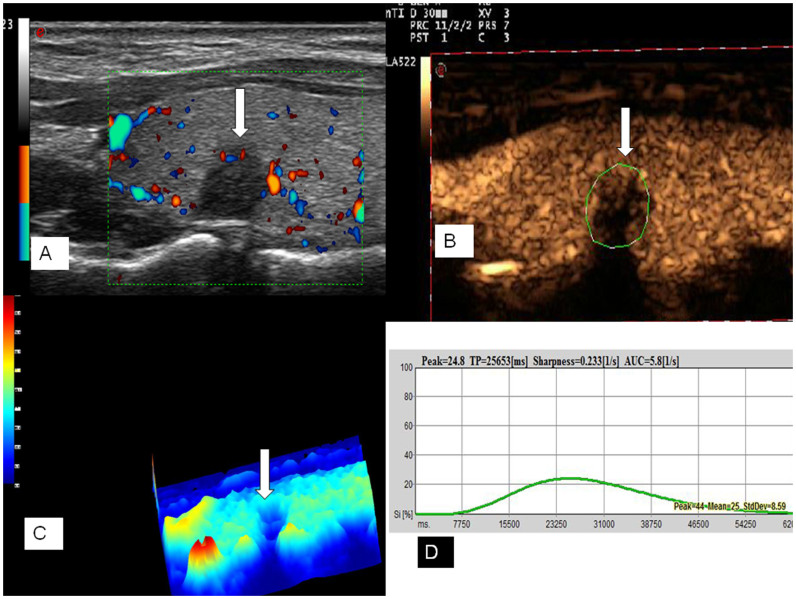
Ultrasound images of a 35-year-old female PTMC patient without CCLNM of the right thyroid lobe. Doppler image showed poor signals in the nodule (A). The CEUS image showed a poor and heterogeneous enhancement (arrow) in the nodule (B). Parametric map showed that the nodule was in blue (arrow), which indicates hypoenhancement with respect to peripheral thyroid parenchyma (C). Numeric values of peak, TP, sharpness, and AUC were automatically calculated based on the time–intensity curve and demonstrated on the top of form (D).
